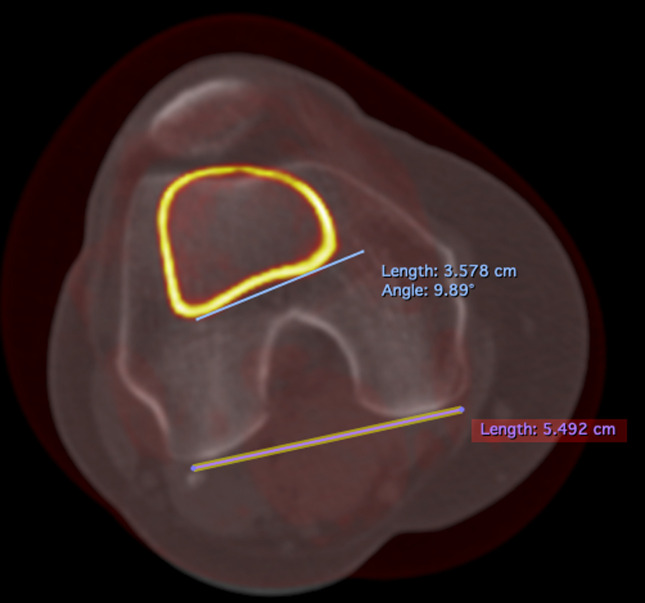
Distal femoral torsion. Superposition of two CT slices. Distal femoral torsion angle is measured as the angle between a line in the posterior cortex at the level of the diaphysis-metaphyseal distal junction and a line joining the posterior borders of both condyles.
